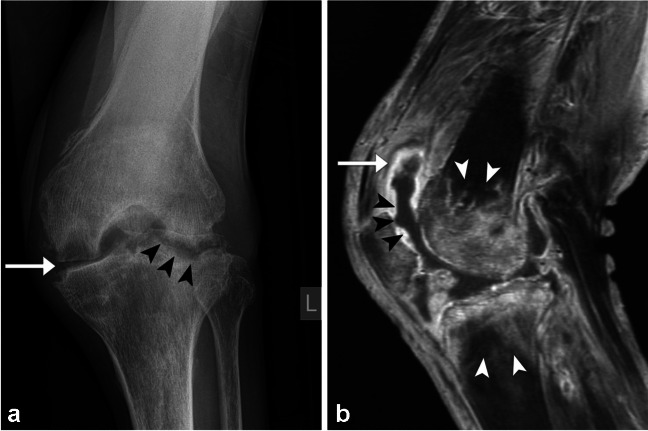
Frontal projection radiograph of the left knee (a) in a 60-year-old male with known septic arthritis demonstrating severe joint space narrowing (white arrow), in addition to periarticular osteopenia and marginal erosions with central erosions (black arrowheads). Sagittal T1-weighted post-contrast MR image of the left knee with fat-suppression (b) in a 79-year-old with an established diagnosis of septic arthritis showing avidly enhancing, thickened synovium (white arrow) with complex, heterogenous joint effusion (black arrowheads). Additional findings of confluent, abnormal marrow enhancement in the distal femur and proximal tibia (white arrowheads) are compatible with concomitant osteomyelitis
